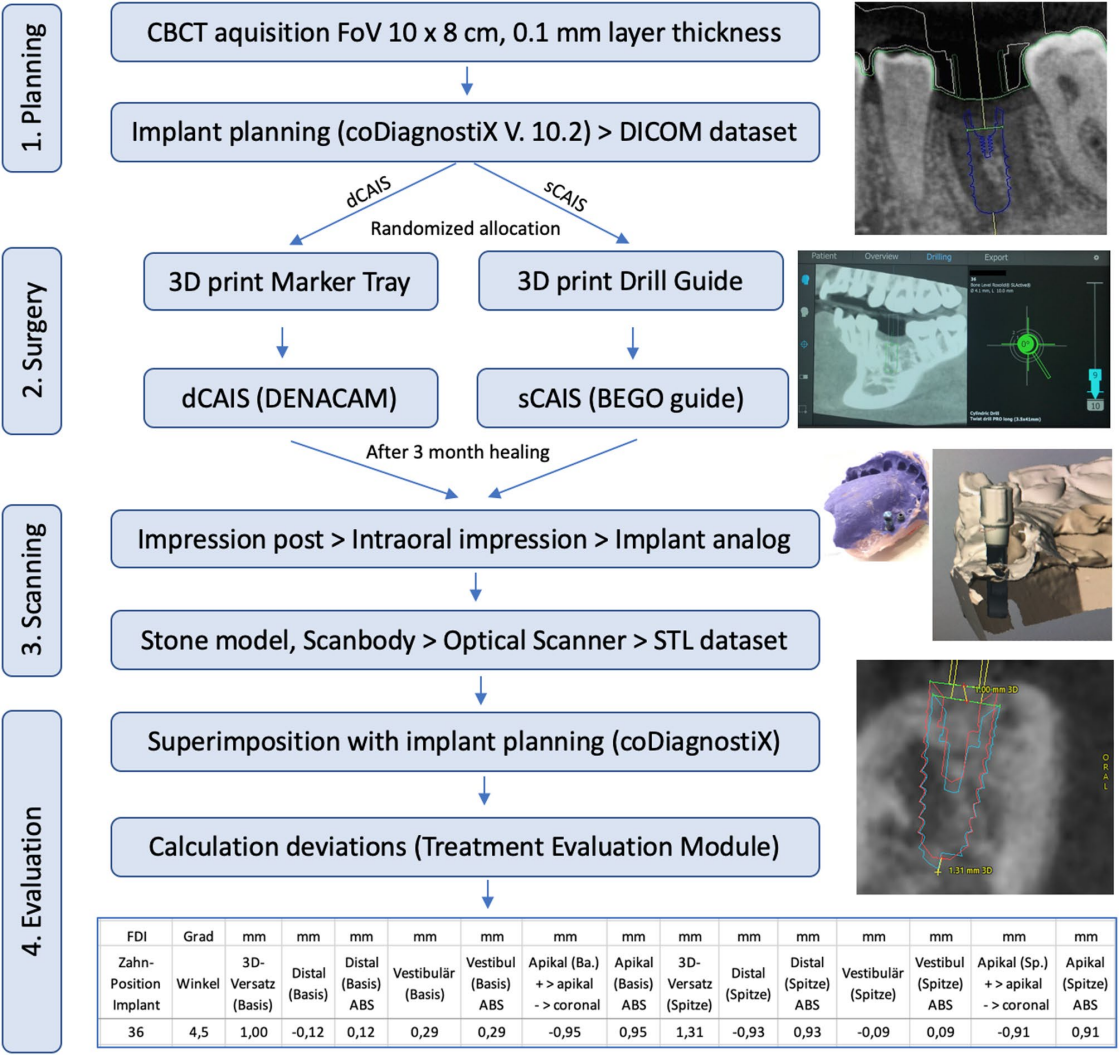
Methodology for measurement of 3D and 2D outcomes (1) Planning: Virtual implant planning (backward planning) in coDiagnostiX, based on CBCT data (DICOM dataset). Patients were randomized to dCAIS (DENACAM) or sCAIS (BEGO surgical guides). (2) Surgery: An STL dataset was generated by segmentation of the CBCT dataset and transferred for 3D printing of the DENACAM marker tray (after virtual planning of marker position) or surgical guide. Execution of CAIS according to drilling protocol. (3) Scanning: After 3 months of healing, an impression post was fixed to the implant, followed by impression taking, fabrication of a stone model with implant analog, and insertion of a scanbody for 3D optical scanning (given is an exemplary scanning image). (4) Evaluation: Superimposition of the scan STL dataset with the original planning data in coDiagnostiX; Calculation of 3D and 2D position/angulation deviations of final implant position compared to planning using the Treatment Evaluation Module (coDiagnostiX).
